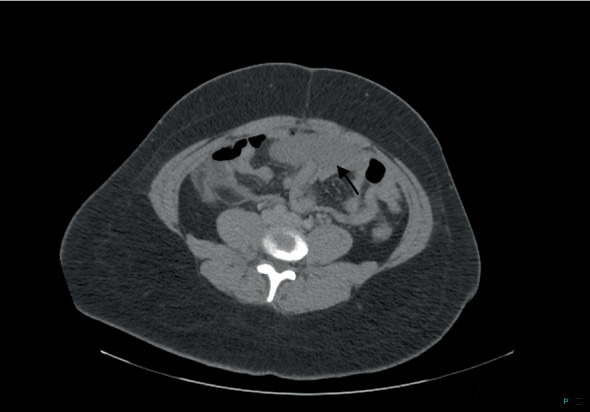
CT image shows a wandering spleen in the lower abdomen indicated by the arrow.
